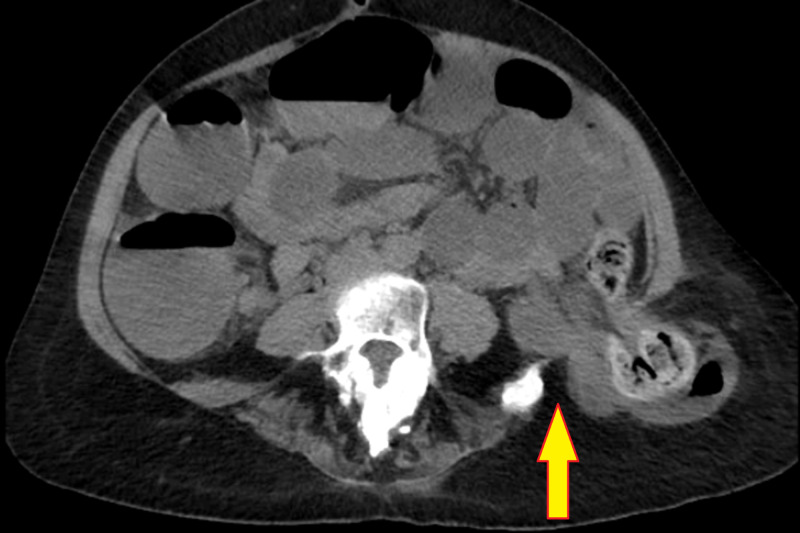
Contrast-enhanced CT shows the herniated colon (arrow) through the iliac bone defect, and a fecalith in the herniated colon.
